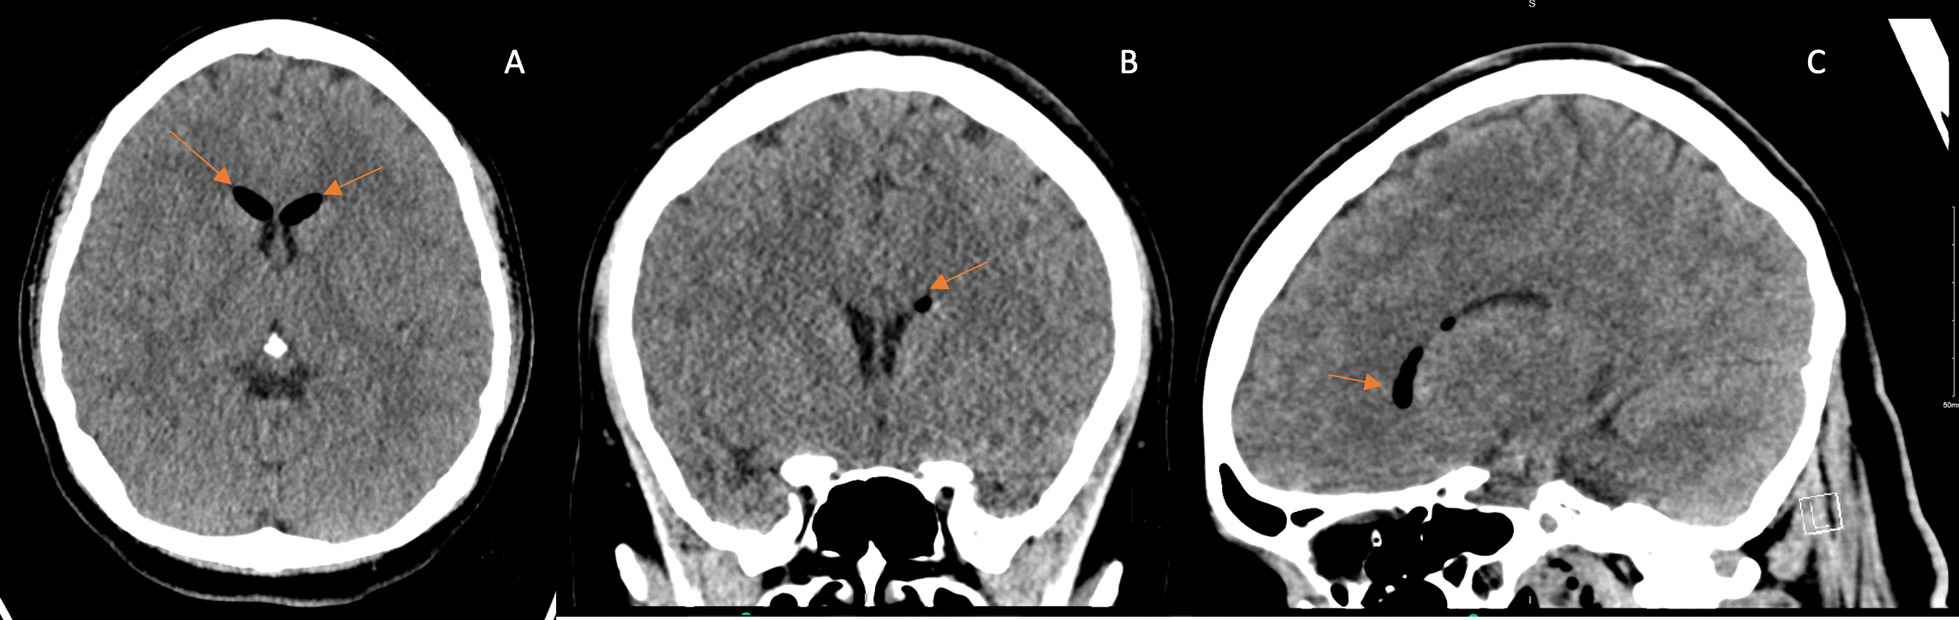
Computed tomography Head on the second presentation showing improvement in the intracranial air. A Axial view, B Coronal view, C Sagittal view. Orange arrows identify the abnormal presence of air
